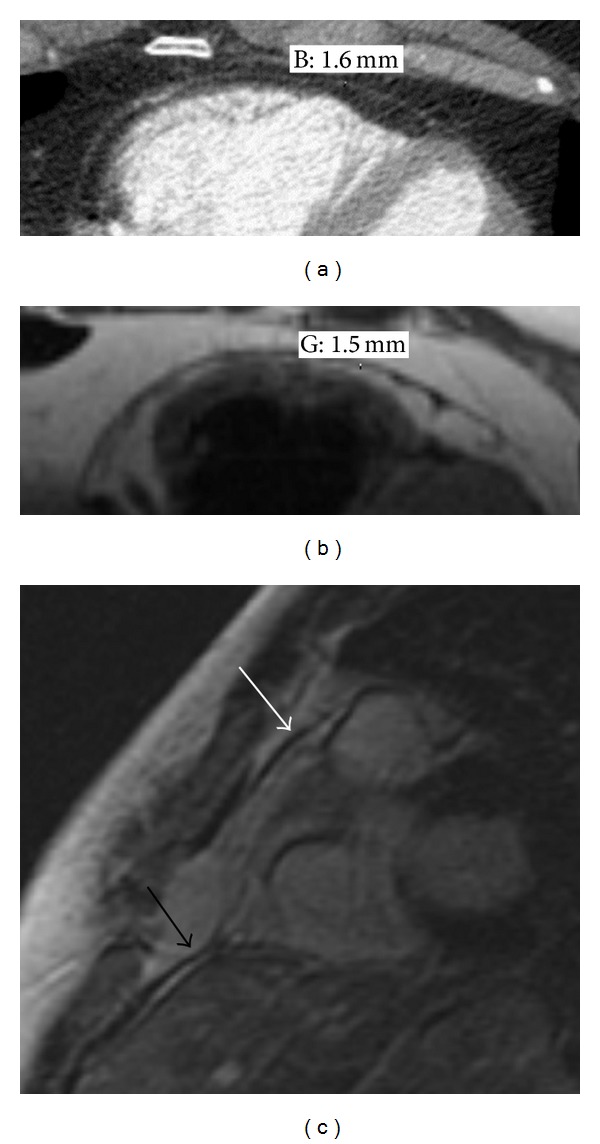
Normal pericardium. (a) Gated contrast-enhanced axial CT and (b) axial double inversion recovery MR images from the same patient show the normal thickness pericardium (parietal and visceral layers indistinguishable) sandwiched between epicardial and pericardial fat layers. (c) A sagittal postcontrast gradient MR image demonstrates both pericardial-diaphragmatic (black arrow) and pericardial-sternal (white arrow) ligaments.
